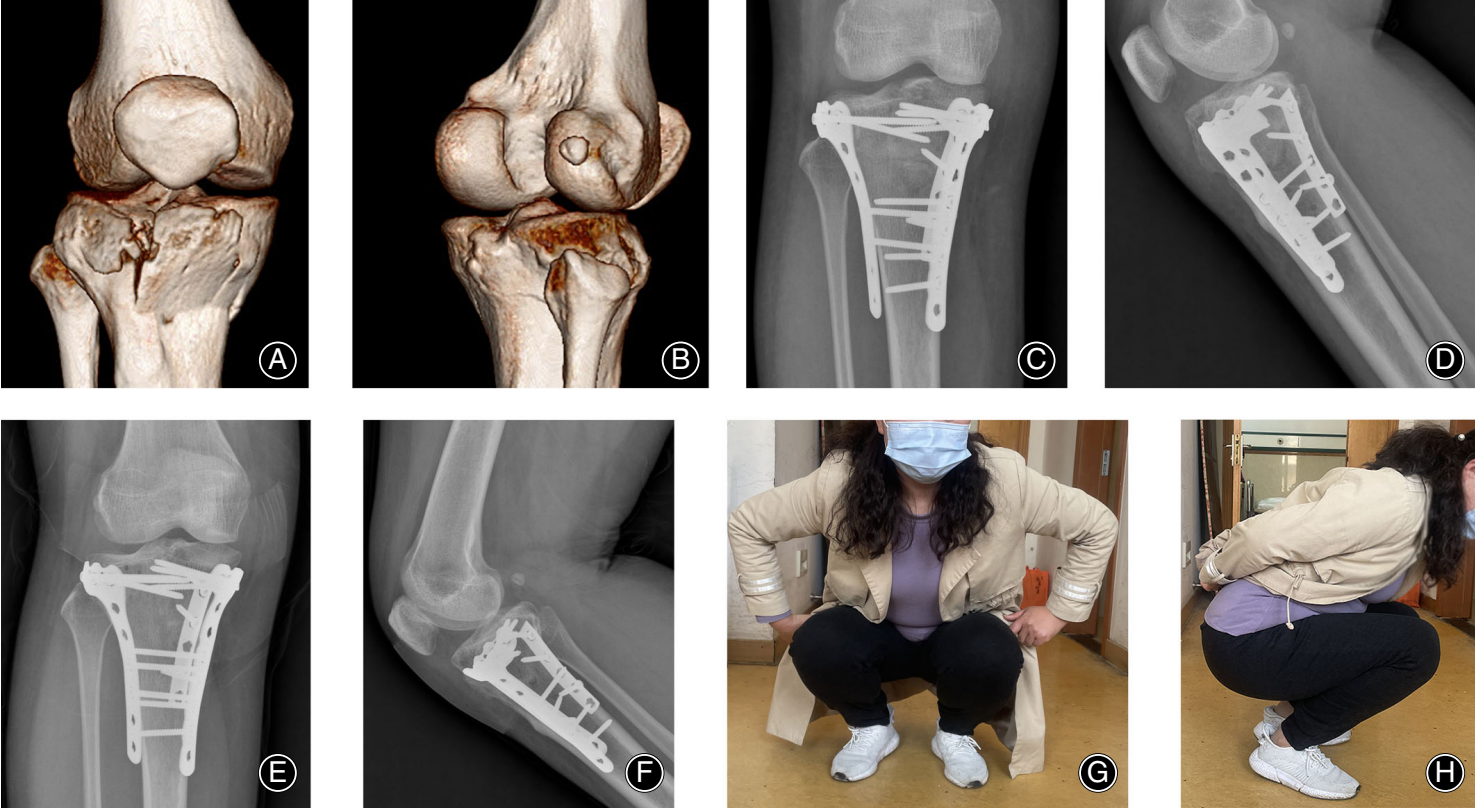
A 41‐year‐old female underwent a motor vehicle accident classified as a hyperextension three‐column fracture and was treated by the triple‐plate method. (A, B) The preoperative three‐dimensional CT image demonstrated that the posterior column was isolated and broken. (C, D) One‐month postoperative X‐rays showed the anatomical reduction was realized by the triple‐plate method. (E, F) A satisfactory anatomical alignment at 6 months. (G, H) Excellent functional knee flexion at final follow‐up (16 months).
